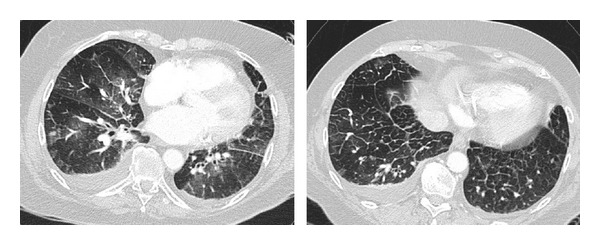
Computed tomography in 77-year-old patients showing signs of congestive heart failure with ground glass opacities, smooth thickening of interlobular septae, and bilateral effusions.
